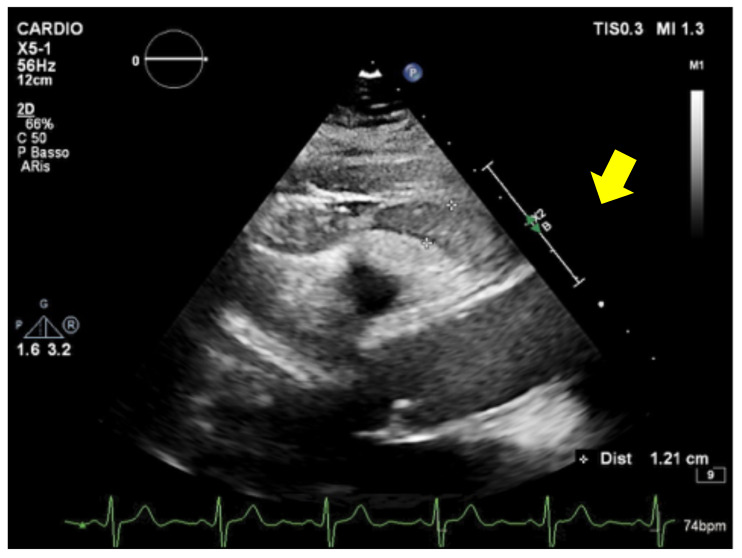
Transthoracic echocardiography showing epicardial adipose tissue. Parasternal short-axis view shows an echolucent layer consistent with epicardial adipose tissue (arrow).
